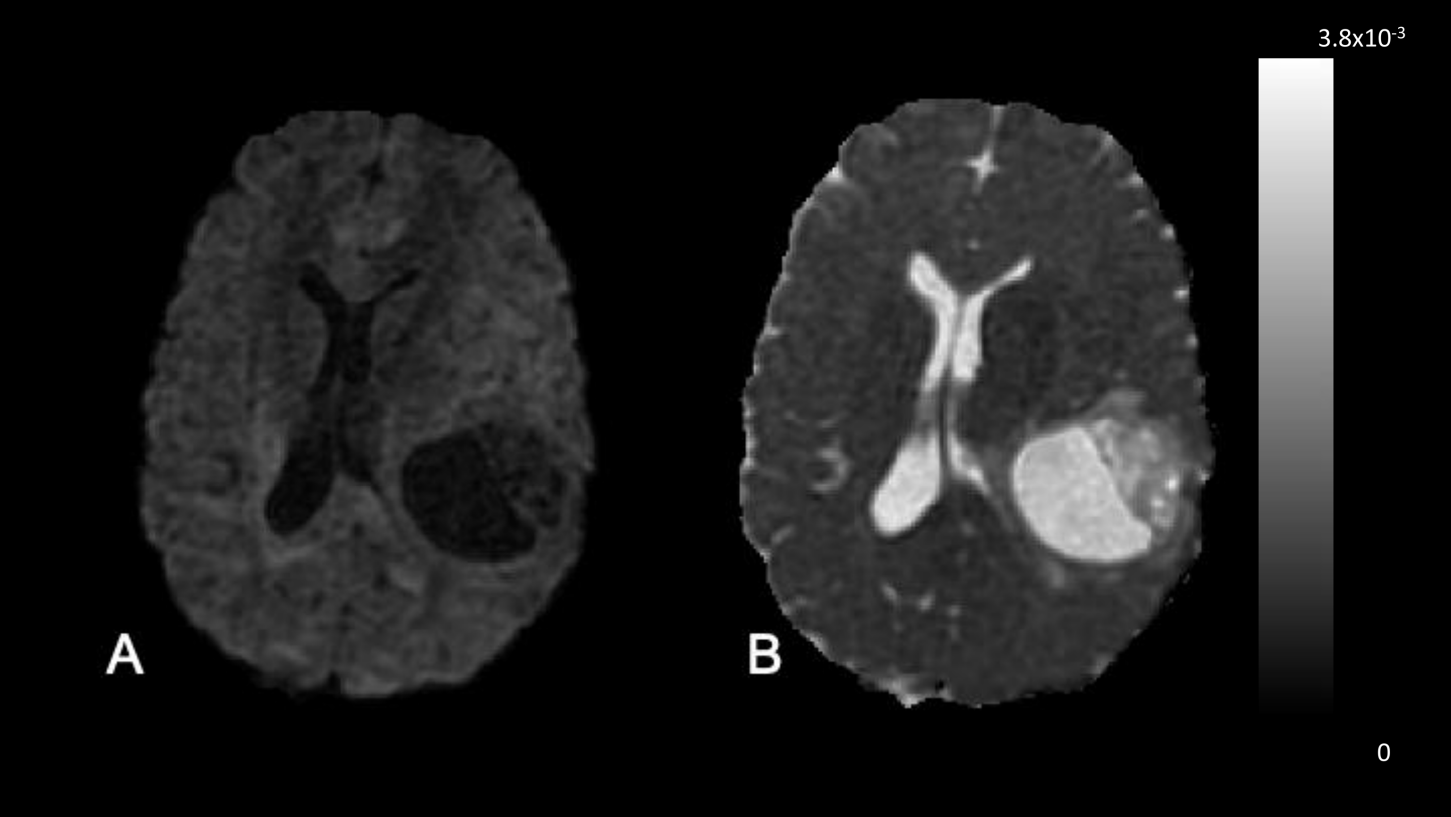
DWI and ADC. These images were acquired in a 50-year-old treatment-naive female patient with glioblastoma, IDH-wildtype. (A) DWI (b = 1000 sec/mm^2^). The necrotic cavity and necrotic rim are DWI hypointense, due to the diffusing spins having moved between the time of the excitation and the refocusing pulse. (B) ADC map. In the ADC map fast diffusion is highlighted by hyperintensity. The combined interpretation of both images gives the qualitative inference that this more likely represents a cavitating or cystic-like tumor with low cellularity rather than a brain abscess or lymphoma.
